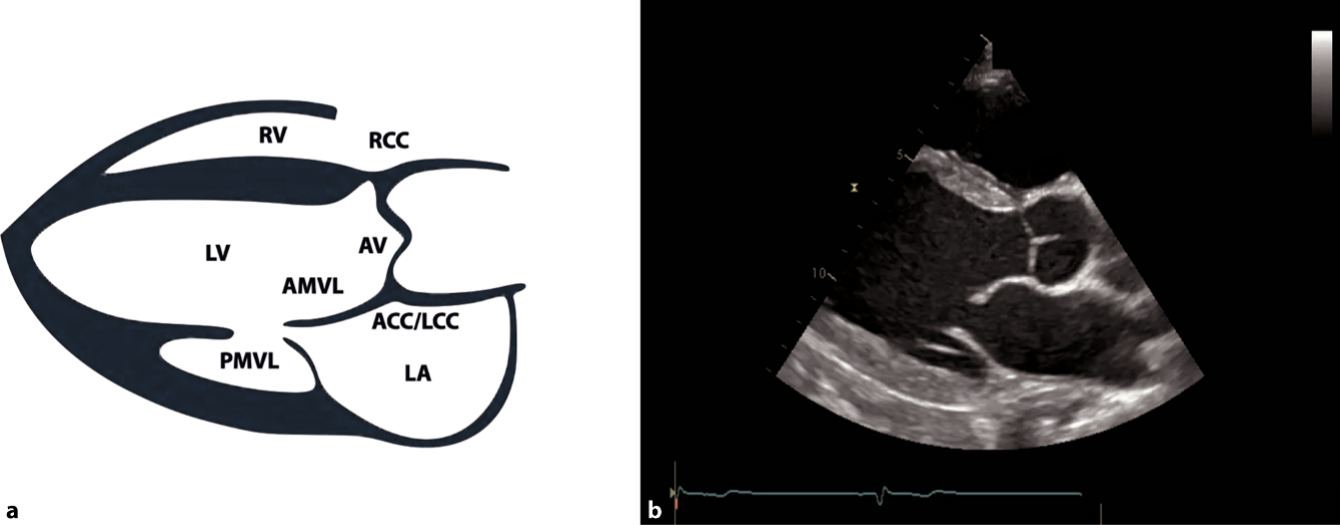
Parasternal long axis view (*PLAX*). a PLAX-left ventricle = *LV*. *AMVL* anterior mitral valve leaflet, *PMVL* posterior MVL, *AV* aortic valve, *RCC* right coronary cusp, *ACC/LCC* non-coronary/left coronary cusp, *RV* right ventricle, *LA* left atrium. b 2D image of a PLAX view
